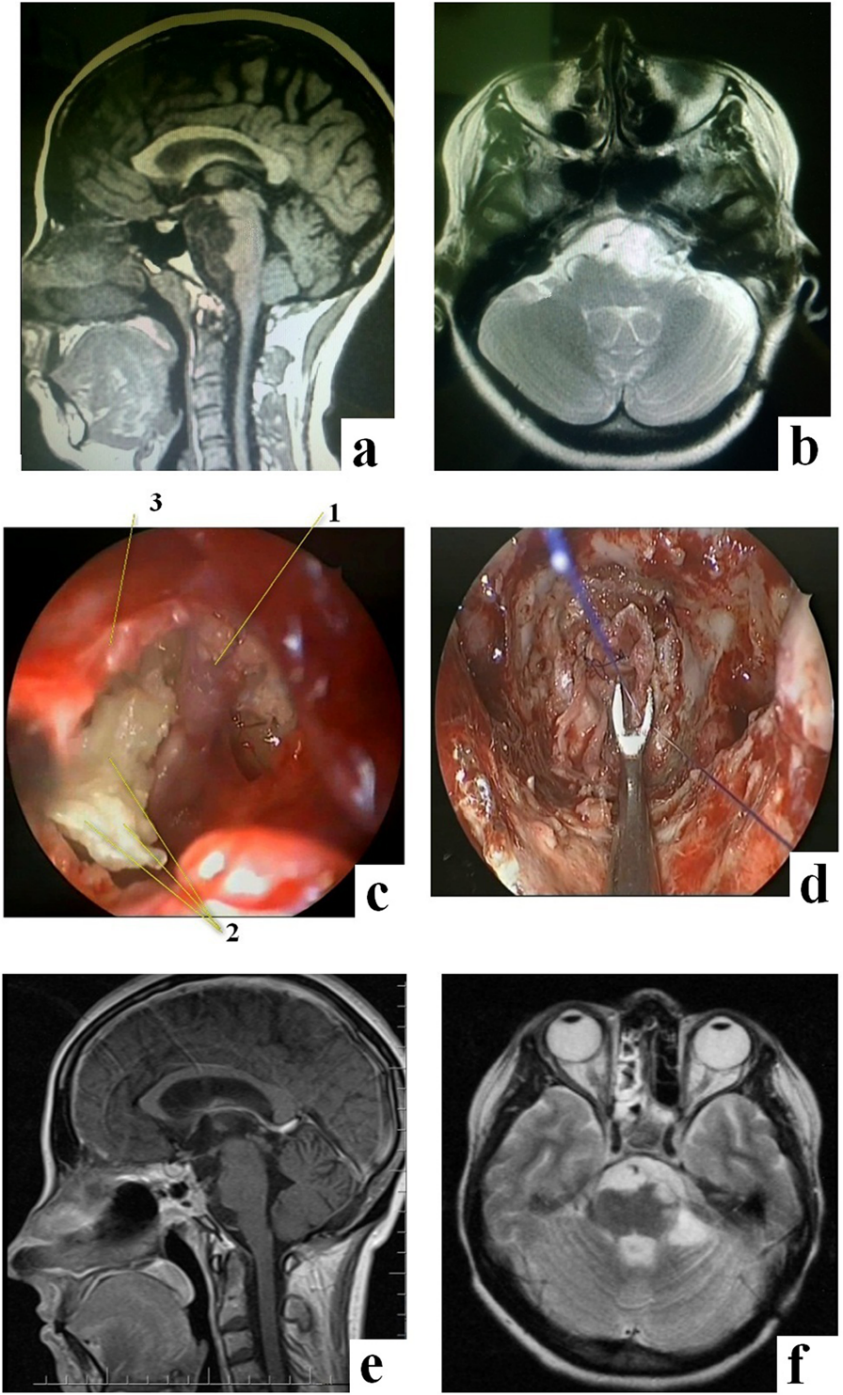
Thirty-four-year-old female patient, with large cholesteatoma of the posterior cranial fossa. a, b MRI before surgery. c Intraoperative photograph: 1—basilar artery; 2—cholesteatoma mass; and 3—dura mater of the clival region. d Intraoperative photograph at the stage of skull base defect reconstruction with microsutures. e, f MRI 4 months after surgery
